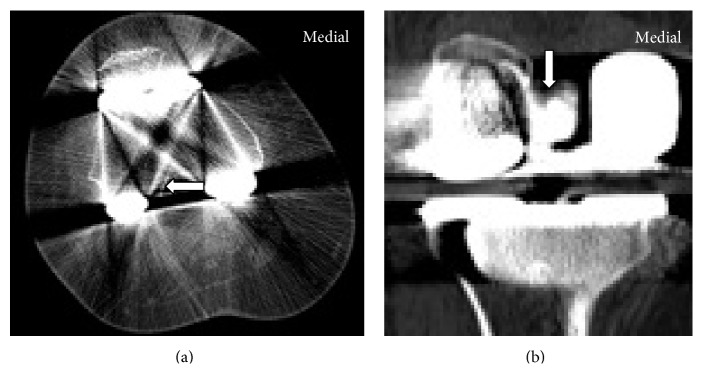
Cement in intercondylar notch (arrow) on CT images.
